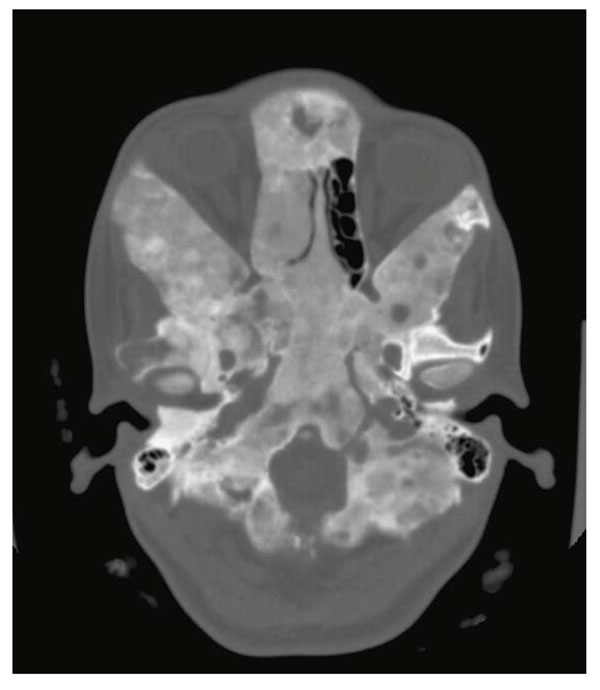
Extensive fibrous dysplasia involvement of the cranial base in a patient with MAS. In patients with PFD or MAS, the anterior cranial base is involved in 95% of the cases as seen in this CT image.
